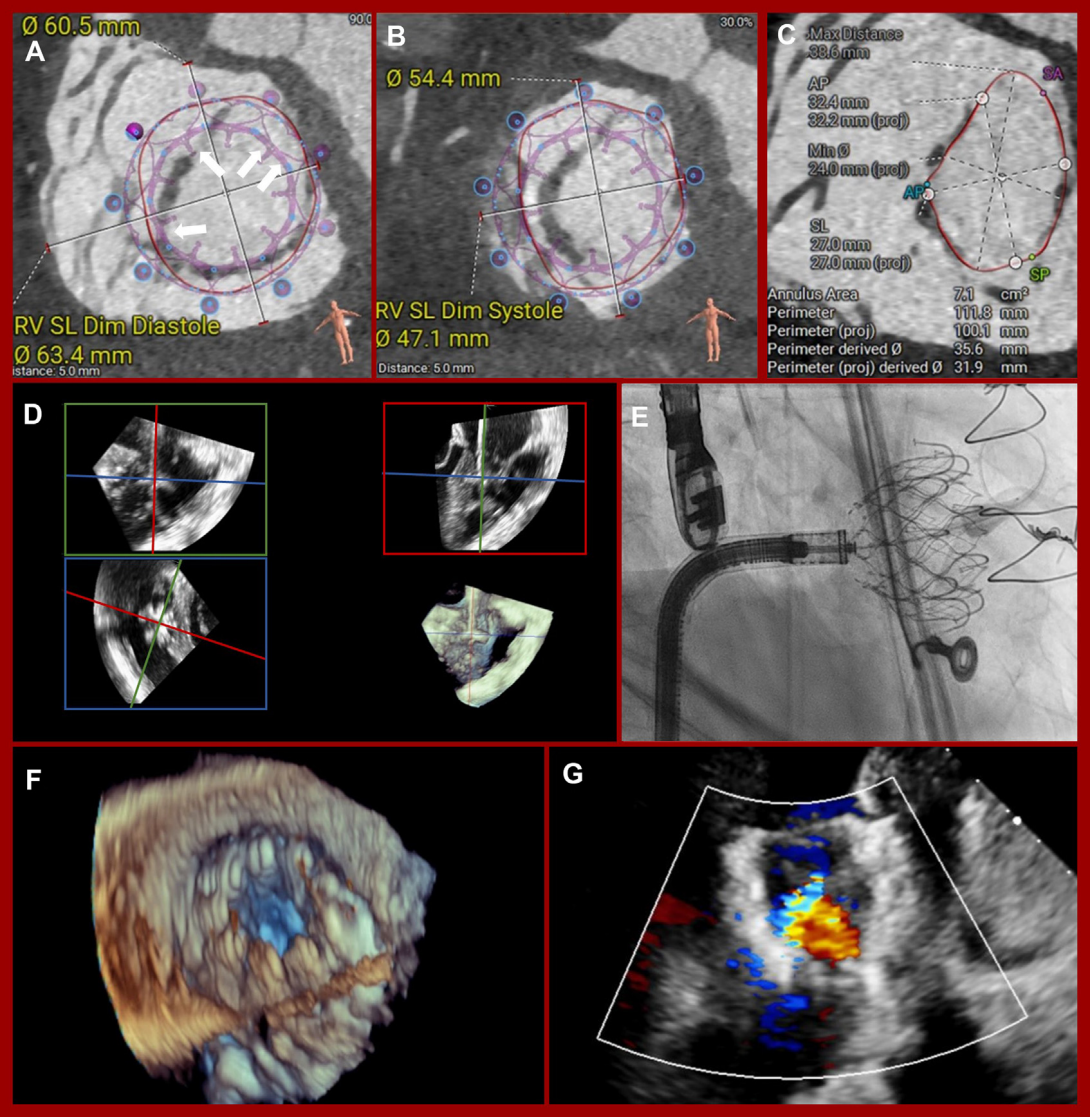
Sizing, Implantation, and Procedural Results of EVOQU (A) Computed tomography sizing of the tricuspid anatomy in diastole. Interruption of the thickened and fused leaflets (open arrows). (B) Computed tomography sizing of the tricuspid anatomy in systole. (C) Tricuspid valve annulus at leaflet tip level. (D) Implantation of the EVOQUE using multiplane reconstruction. (E) Full atrial deployment. (F) Stable postinterventional position. (G) Mild valvular tricuspid regurgitation.
